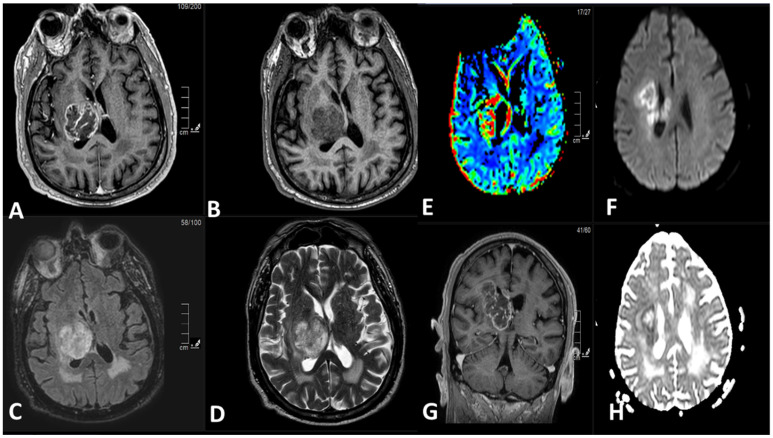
(A) Axial T1-weighted post IV contrast sequence. (B) Axial T1-weighted pre-IV contrast sequence. (C) Axial FLAIR sequence. (D) Axial T2-weighted sequence. (E) Dynamic susceptibility contrast (DSC) MR perfusion images, cerebral blood volume (CBV). (F) Diffusion weighted images (DWI), b 1000. (G) Coronal T1-weighted post IV contrast sequence. (H) Apparent diffusion coefficient (ADC) map.
